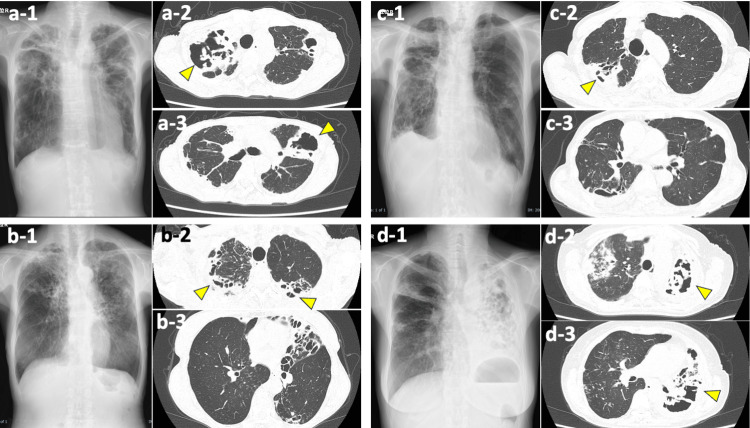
Radiographic Profiles of Pulmonary Manifestations. (a-1), (b-1), (c-1), and (d-1) depict the anterior-posterior chest radiographs, while (a-2,3), (b-2,3), (c-2,3), and (d-2,3) display the axial computed tomography (CT) scans of four distinct patients, denoted as (a), (b), (c), and (d), respectively. Arrowheads in the images highlight the presence of cavitary lesions within the pulmonary parenchyma.
